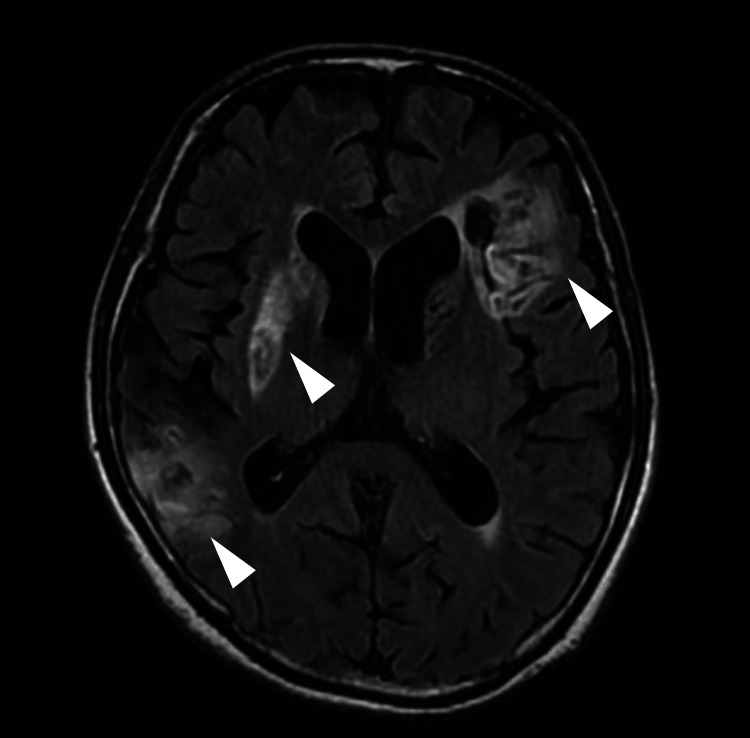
MRI-Fluid-Attenuated Inversion Recovery (FLAIR) images of the head showing cerebral infarction of the bilateral cerebral hemisphere The patient was diagnosed with a right hemispheric cerebral infarction due to occlusion of the right middle cerebral artery. His medical history included a left cerebral infarction. The patient presented with pseudobulbar palsy due to the bilateral cerebral hemispheric lesions (arrowheads).
